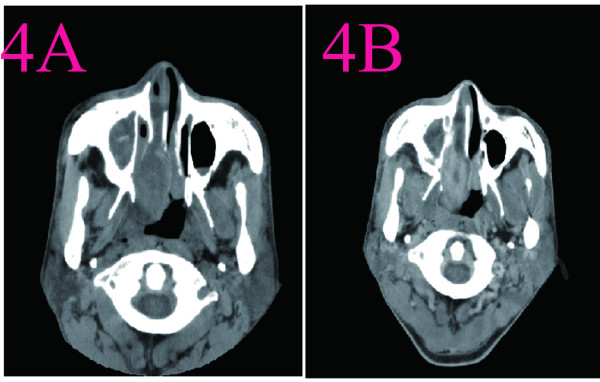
CT scans of nasal cavity in Case 3.A: CT scan showed that a well-defined large expansile soft-tissue mass in the left nasal cavity from choana to the inferior turbinate. B: There was patchy enhancement on contrast CT.
